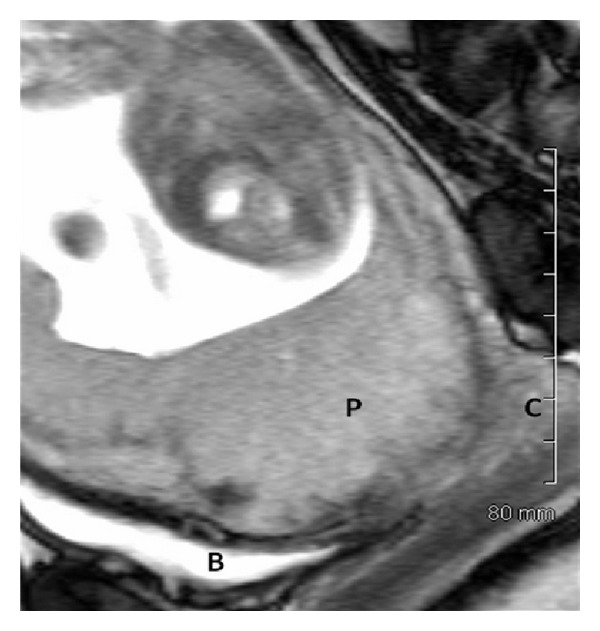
Magnetic resonance imaging (MRI) at 17 weeks' gestation demonstrating focal disruption of the anteroinferior myometrium with placental invasion. P: placenta, B: bladder, and C: cervix.
